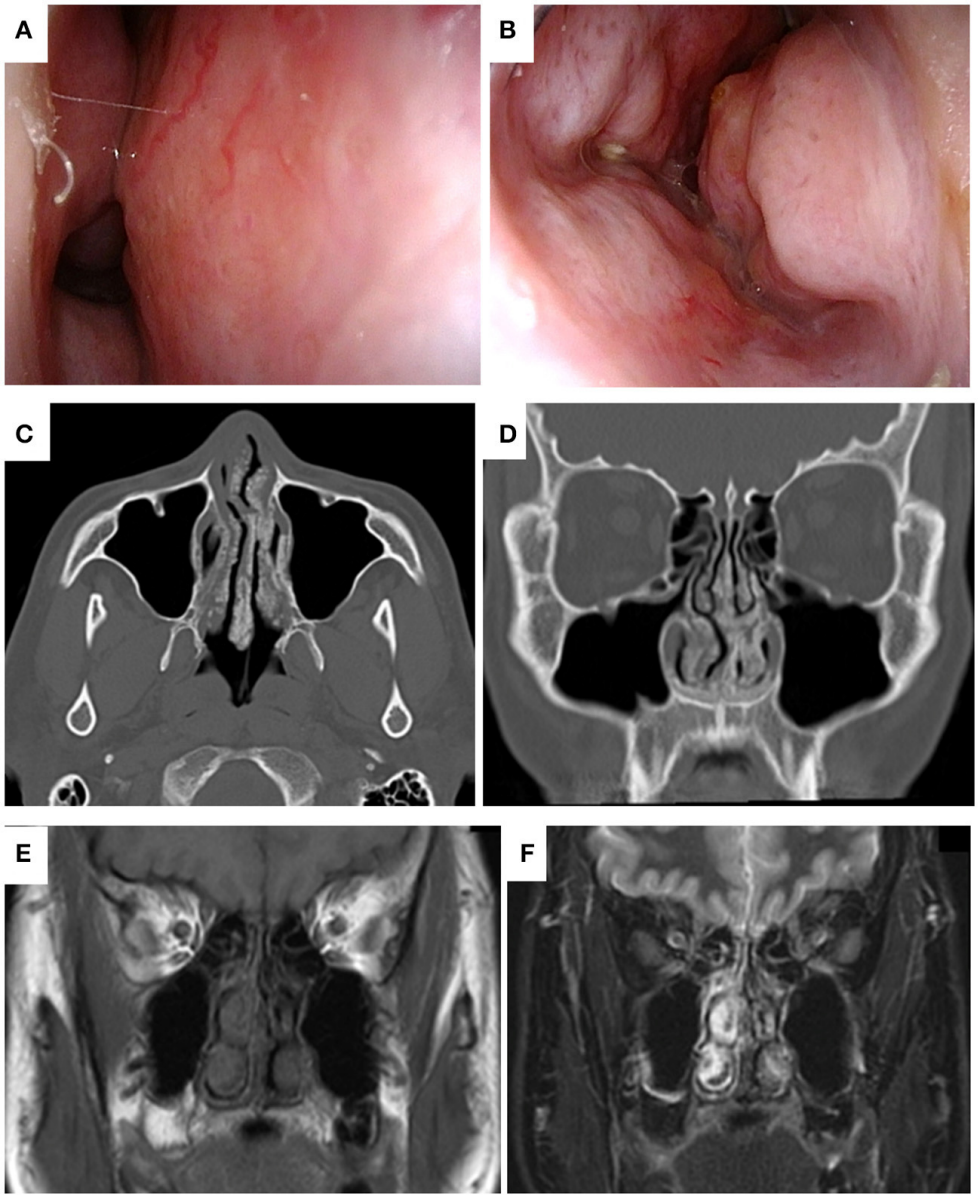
Endoscopic findings of localized sinonasal amyloidosis viewed from the right (A) and left (B) nasal cavities at the first visit to our hospital. The deviation of nasal septum to the right. The mucosa was smooth and pink, with nodular thickening, and bilateral narrowing of the common nasal meatus was observed. Plain computed tomography (CT) of the present case obtained at the previous hospital. Axial (C) and coronal (D) views of bone window CT of the head showed the deviation of the nasal septum to the right, thickening of the bilateral nasal mucosa, and deposition of microcalcified material in the submucosa of the nasal cavity, including the bilateral nasal septum, and middle and inferior turbinates. Magnetic resonance images of the present case obtained at the first visit to our hospital. Coronal views of plain T1-weighted (E) and T2-weighted imaging (F) of the head showed bilateral thickening of the nasal mucosa and numerous hypointense punctate signal areas in the submucosa of the entire nasal cavity.
